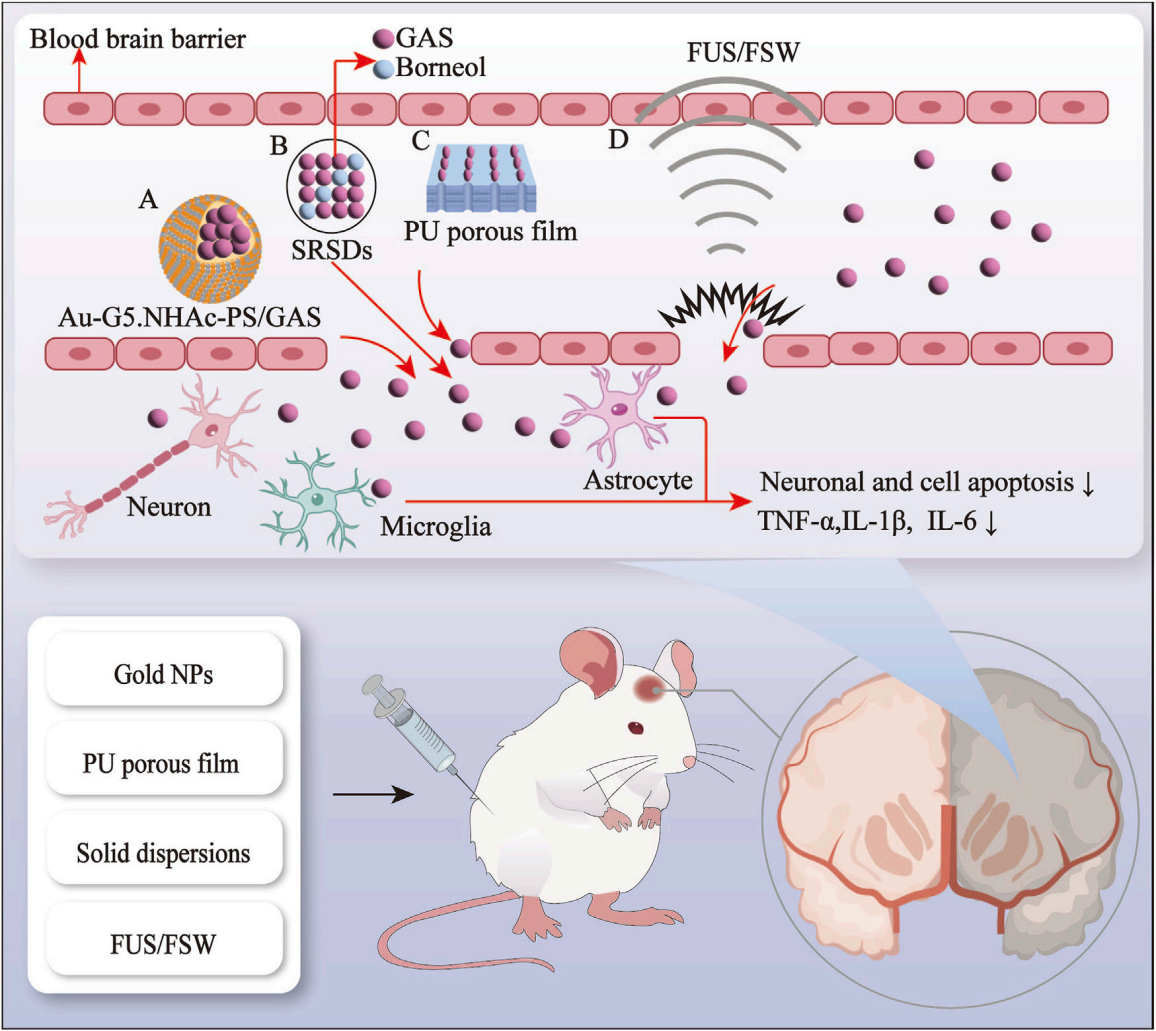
The gastrodin drug delivery system and physical enhancement methods via the blood-brain barrier route. A, gold nanopaticles; B, solid dispersions, C, polyurethane porous film; D, focused ultrasound and focused shockwave.
